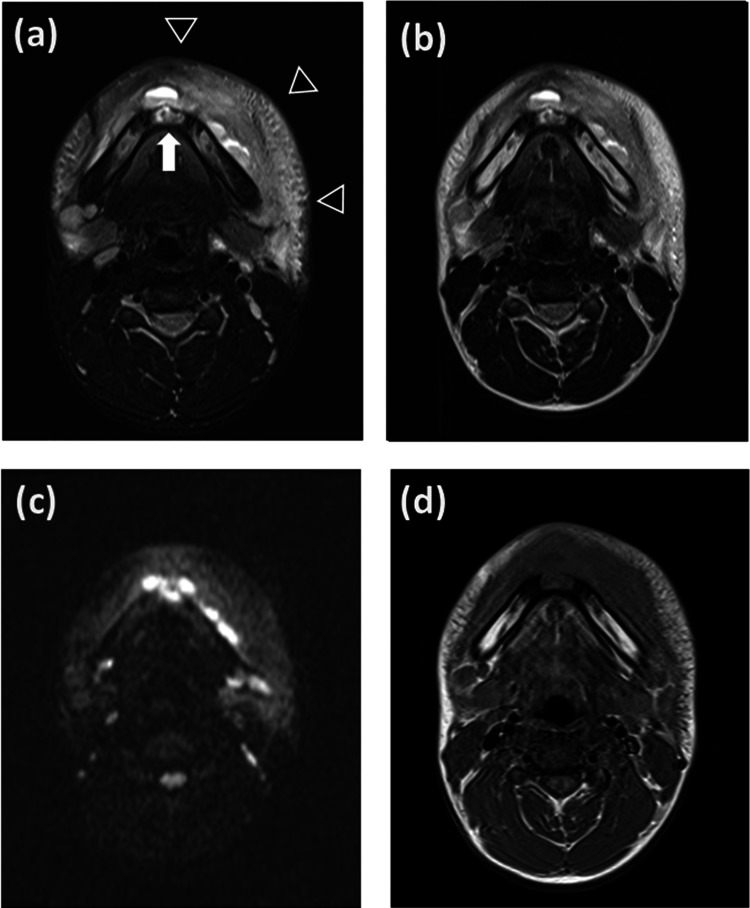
Magnetic resonance imaging. (a) Fat-suppressed T2-weighted imaging. An arrow indicates an area of high signal intensity in the middle of the mandible. Arrowheads indicate severe swelling of the subcutaneous tissue of the jaw and fluid retention along the mandibular bone. (b) T2-weighted imaging. (c) Diffusion-weighted imaging. (d) T1-weighted imaging.
